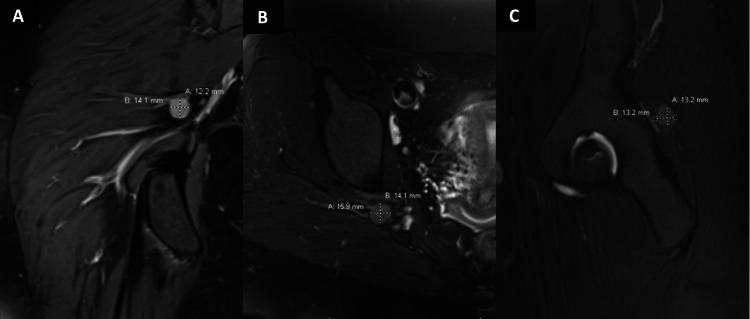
Case 2 preoperative imaging Patient's preoperative MRI of the right leg shows an approximately 1.4cm x1.2cm x 1.5cm contrast-enhancing lesion (A: coronal) that is isointense on T2 fast-saturated imaging (B: axial & C: sagittal).
